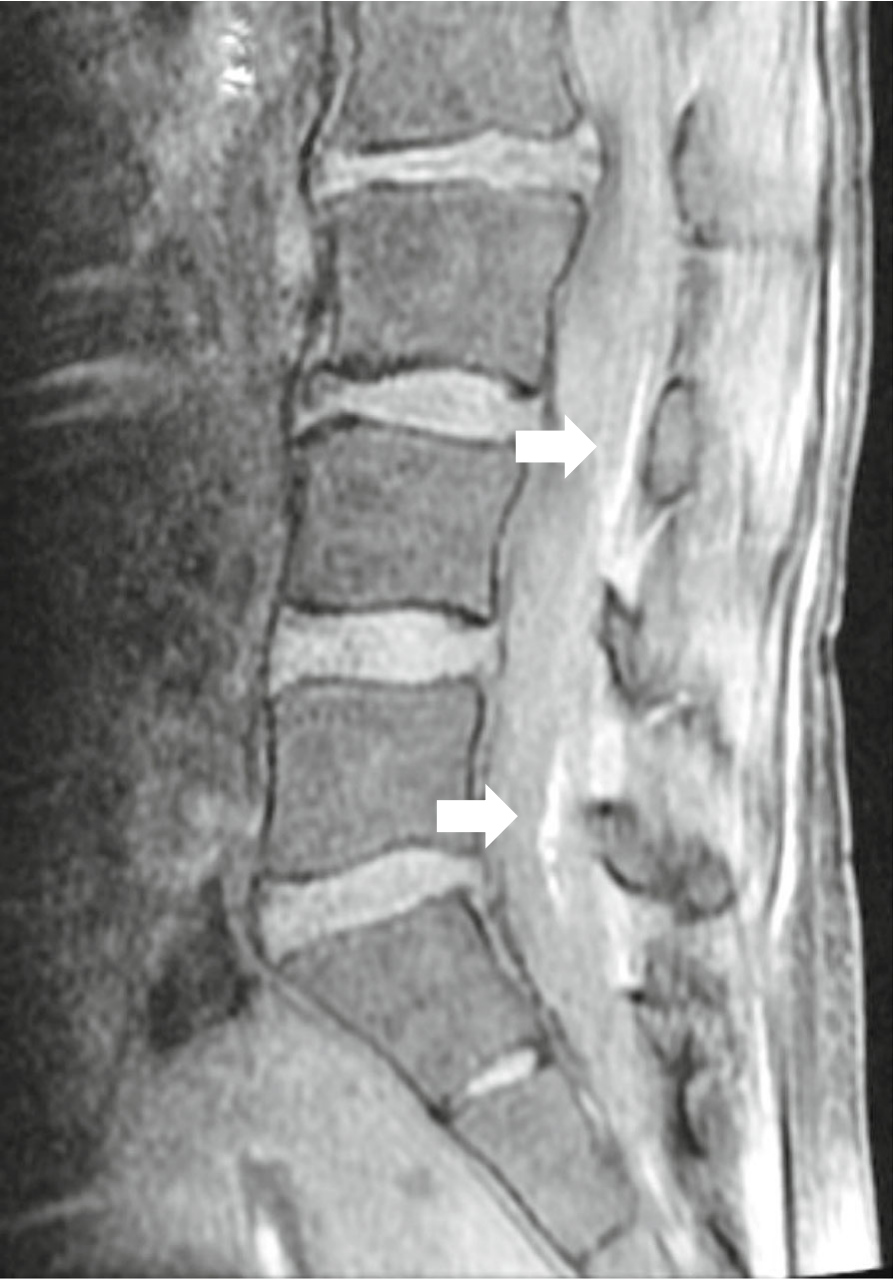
A parasagittal T1-weighted image. The magnetic resonance image shows subdural blood collection from the epidural blood patch. White arrows indicate intrathecal blood. These subdural clots were identified as two separate hematomas on MRI
